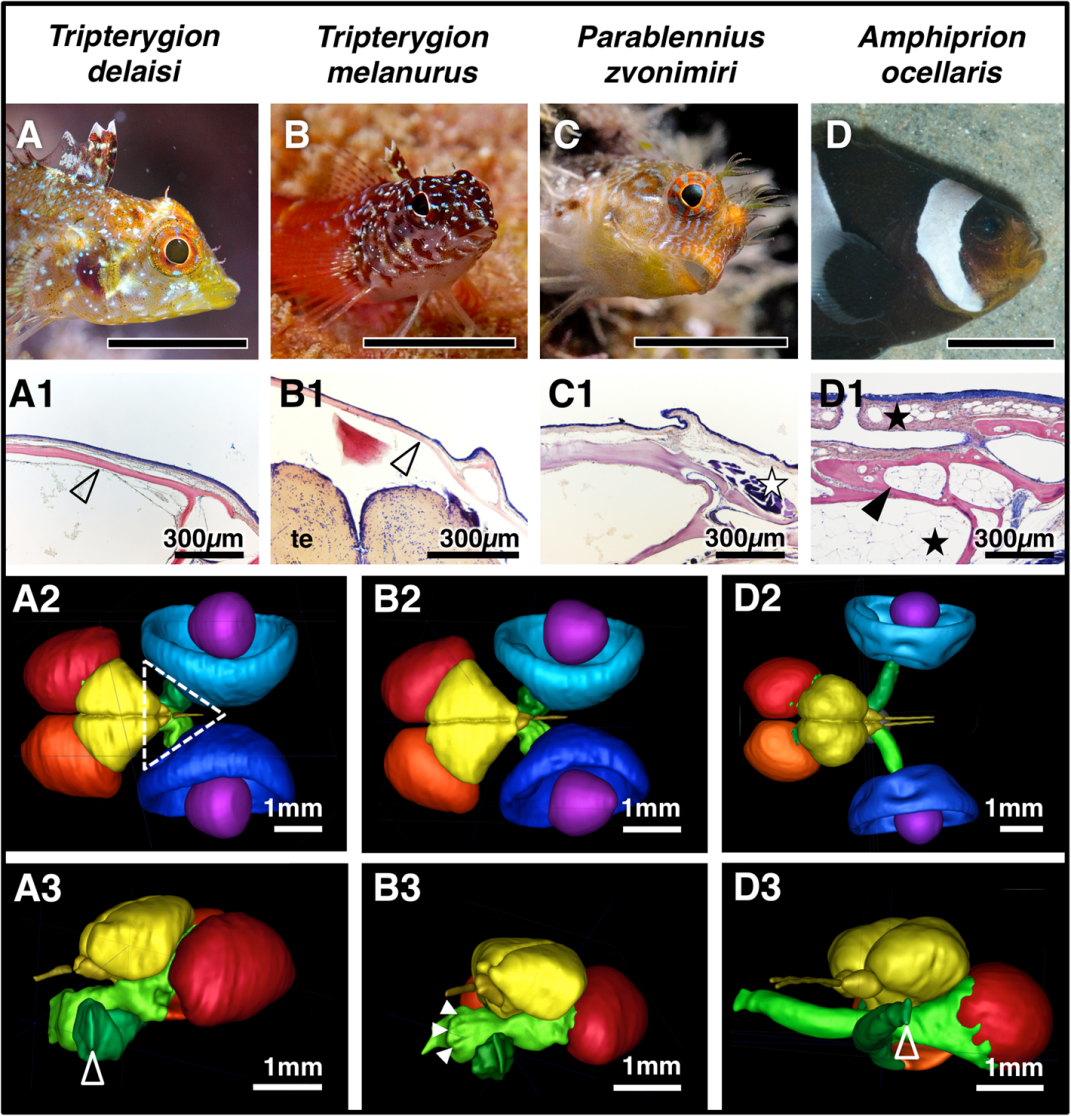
Skull structure and visual system of *T. delaisi* (a), *T. melanurus* (b), *P. zvonimiri* (c), and *A. ocellaris* (d; *scale bars* 1 cm). a1-d1 Close-up of the dorsal skull and dermis. Triplefins have delicate but solid bones (a1-b1, *open arrowheads*; *te* telencephalon) compared to the thicker, chambered bones (d1, *black arrowhead*) of the clownfish. The skull is only covered by a thin dermis in triplefins, while the blenny has muscles running between the dermis and the skull (c1, *white star*), and the clownfish has fatty tissue (d1, *black stars*) throughout its head. a2-d3 MRI segmentations of inner head anatomy in *T. delaisi*, *T. melanurus*, and *A. ocellaris:* retinae (*blue*), lenses (*purple*), ONs and tracts (*green*), optic tecta (*red*), telencephalon, olfactory nerves and bulbs, and diencephalon (*yellow*). *T. delaisi* (a2, a3) and *T. melanurus* (b2, b3) are practically identical, except for differences in absolute size. a2-d2 Dorsal view. The *dashed triangle* frames the exposed part of the ON, which coincides with the area of most efficient ONT eyeshine induction (see Fig. 4a). In *A. ocellaris* (d2, d3), the telencephalon covers a smaller part of the nerves, but these lie deeper in the head. a3-d3 Frontolateral view onto the left optic disc. While narrow and elongated in triplefins, it is shorter and elliptical in the clownfish (*open arrowheads*). The ridges along the nerve surface in b3 (*solid arrowheads*) result from the triplefins’ loose ON pleats. The clownfish’s ON is smooth in comparison, due to the denser pleating
